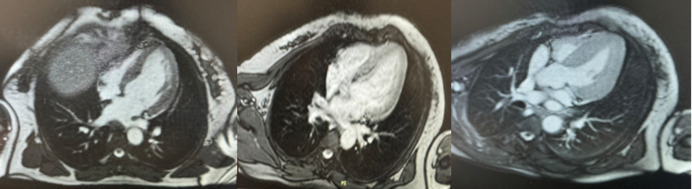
cardiac magnetic resonance imaging revealing hypokinesia of the apical and mid-segments of the inferior and inferolateral wall of the left ventricle, without evidence of infarction
